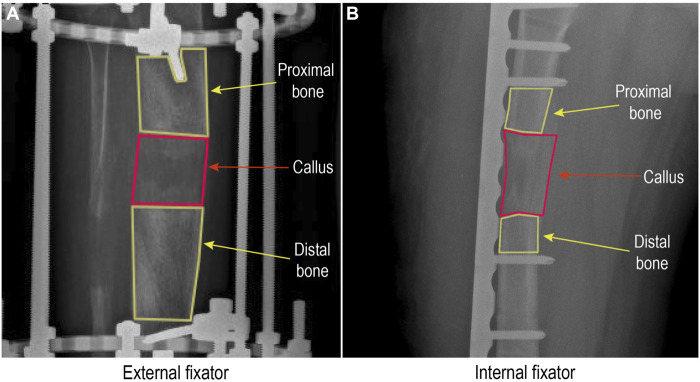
Pixel value assessment from a radiograph in a picture archiving and communication system.
